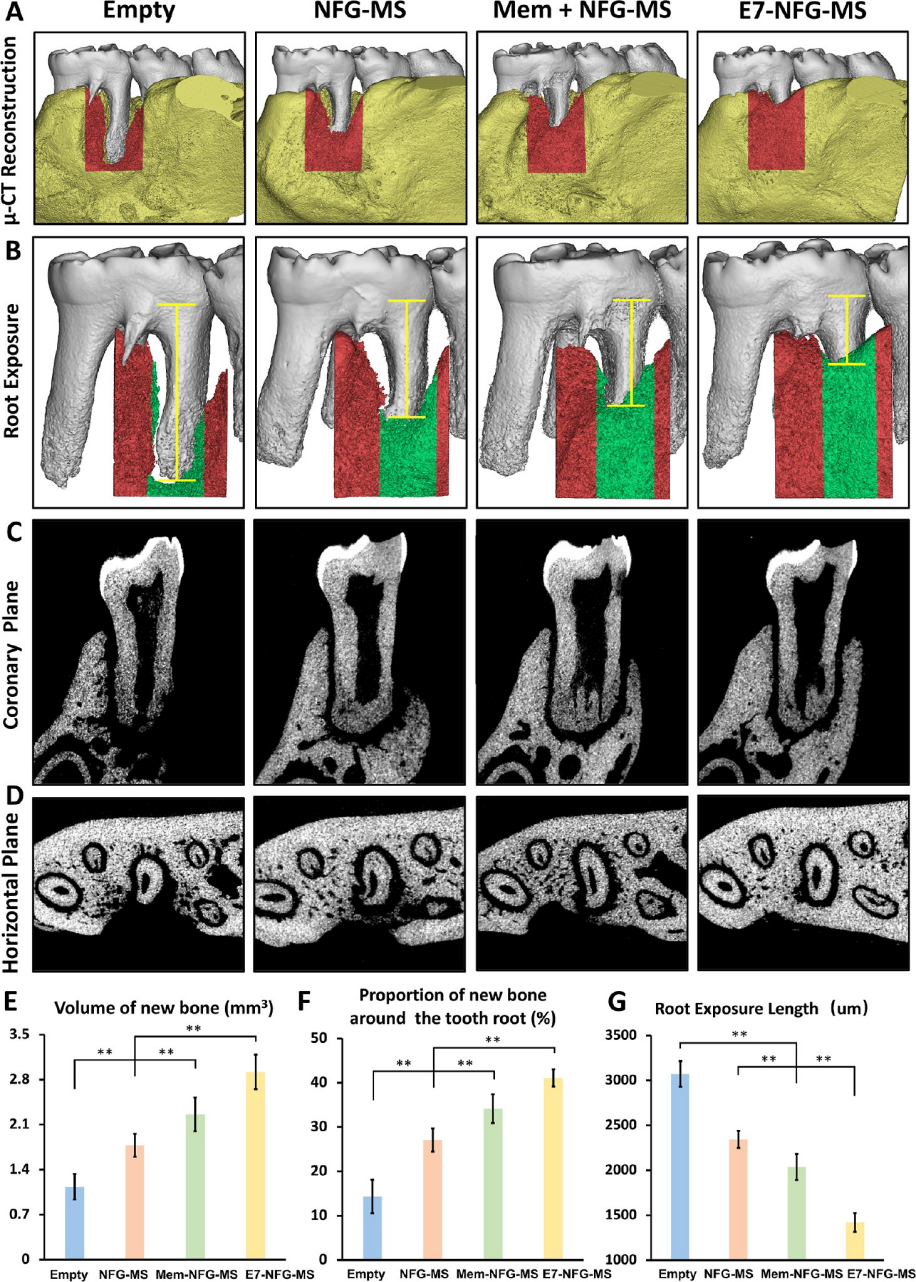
μ-CT analysis of newly formed alveolar
